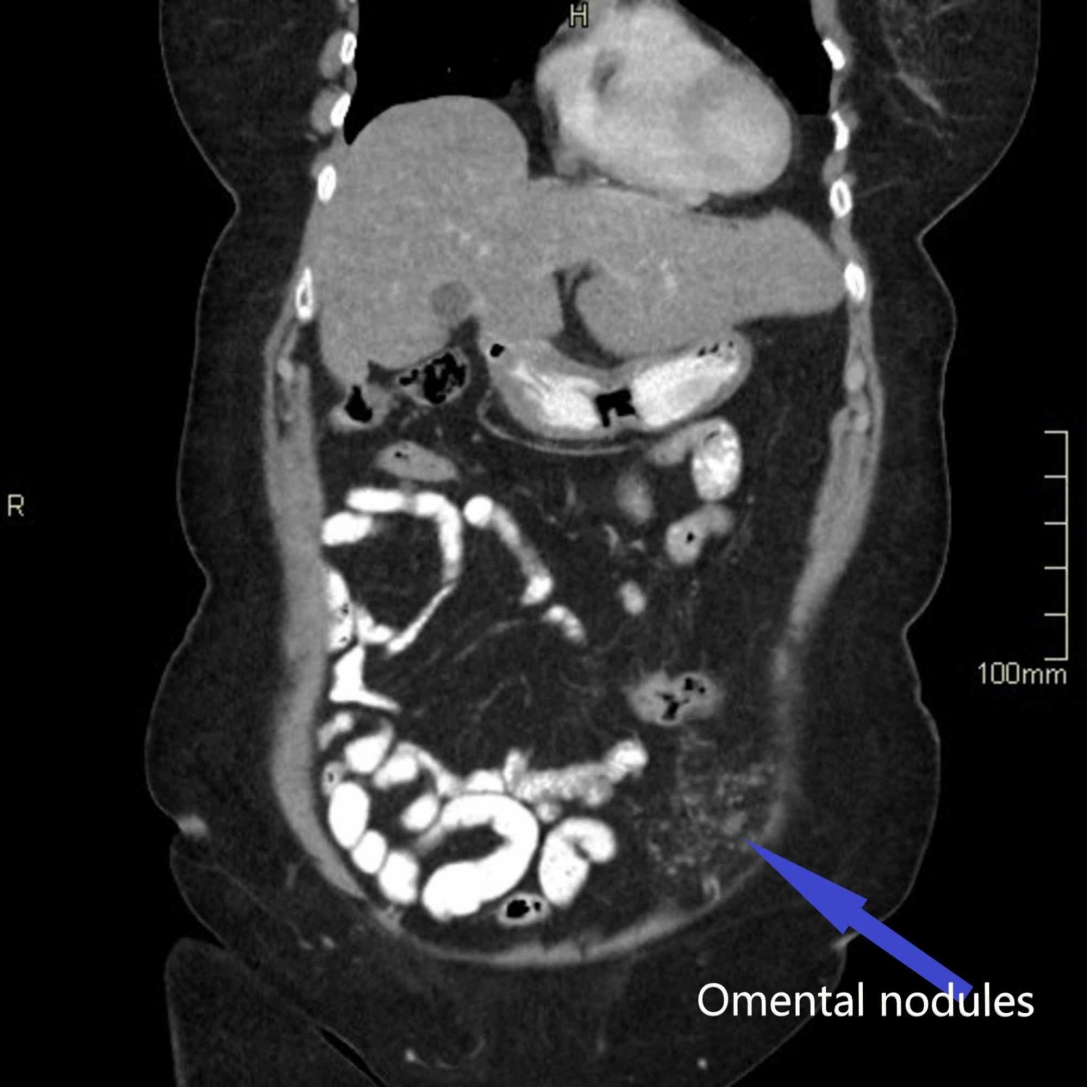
Coronal view from CT scan
